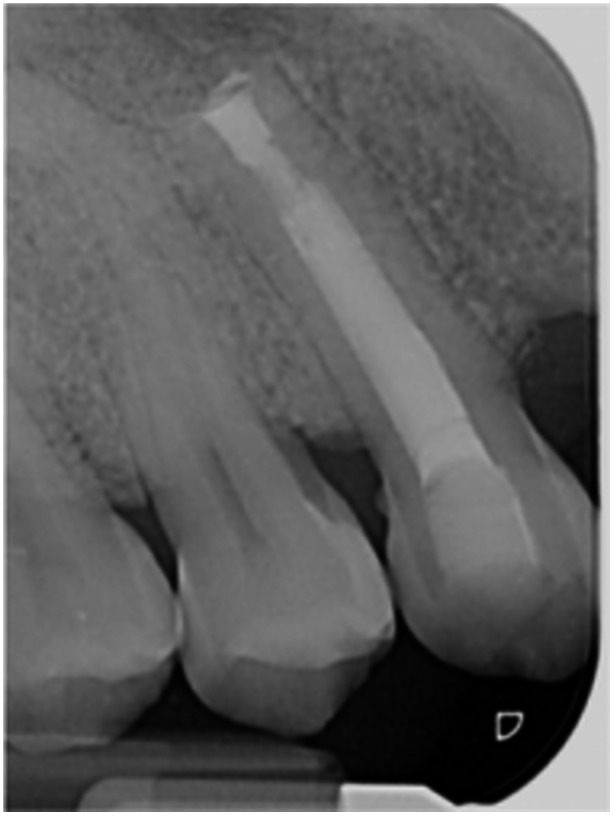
Case 8: The preoperative CBCT and periapical radiograph show an extensive periapical radiolucency associated with tooth 13, which has thinned the labial and palatal cortical plates. Apical surgery was performed on tooth 13. At 6 months, complete healing is evident.
